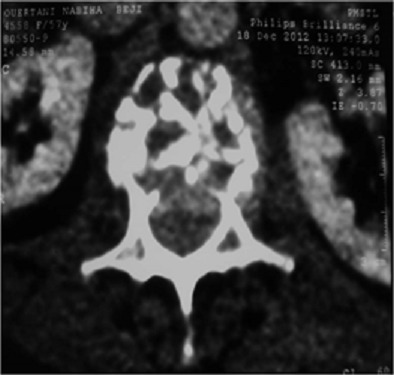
Axial computed tomography image demonstrating heterogeneities in the body of L2 with tumoral epiduritis
